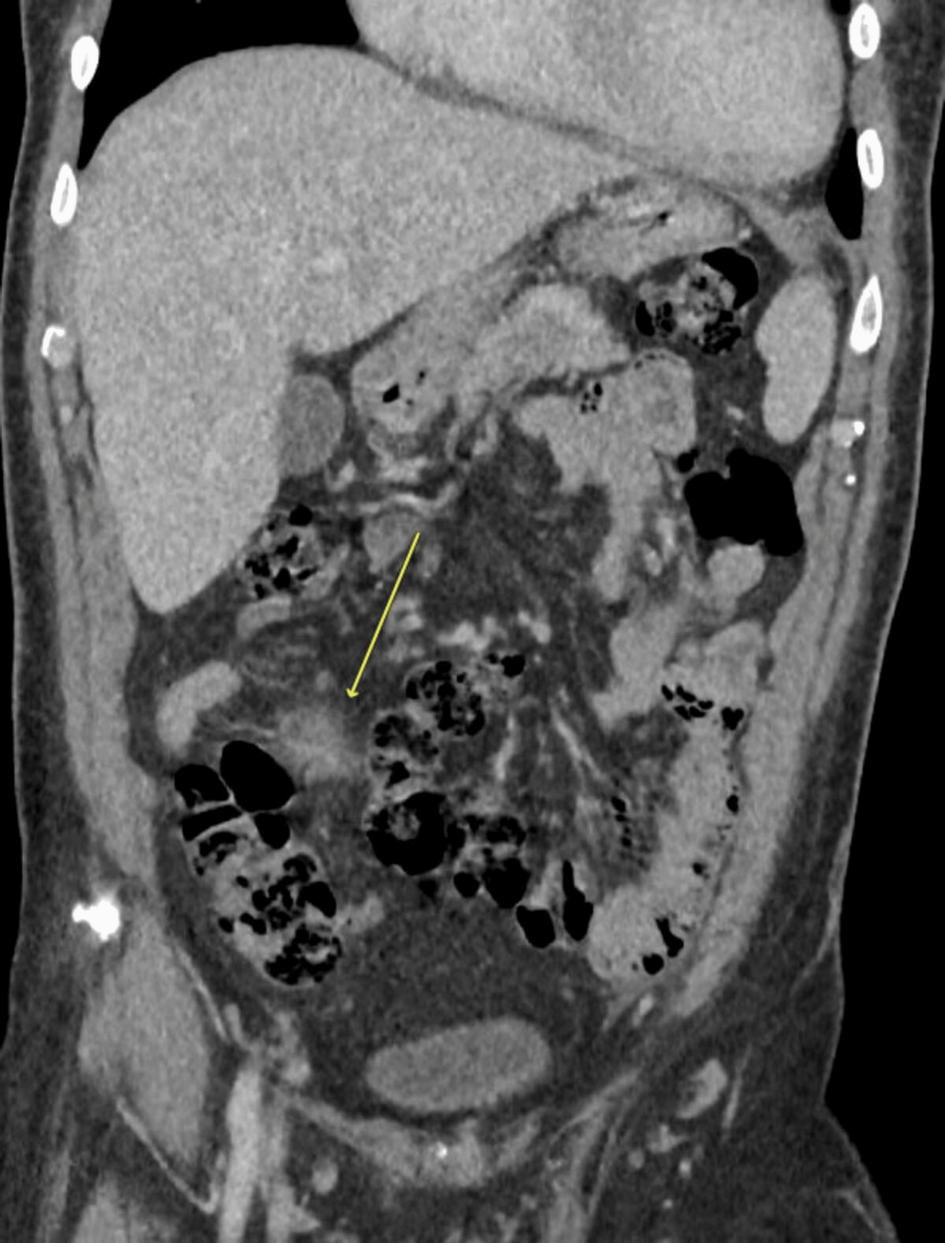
Abdominal CT scan with contrast, coronal view, showing a small soft tissue inflammatory lesion with stranding of the adjacent fat planes (arrow), with the impression of an inflammatory lesion (panniculitis).
